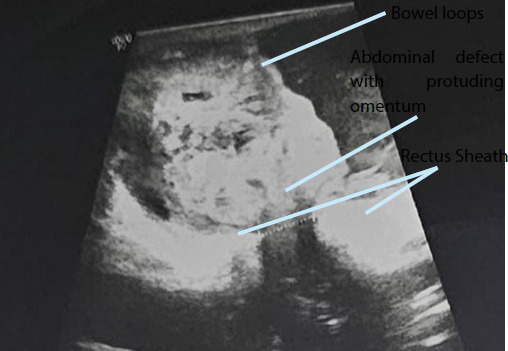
Ultrasonographic image showing a defect in the umbilical region with herniated bowel loops.
